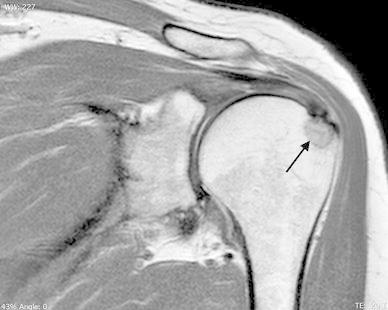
T1-weighted coronal oblique MRI highlights a greater tuberosity osteolysis (*black arrow*) in a case with a calcium deposit of the SS in contact with the bone
